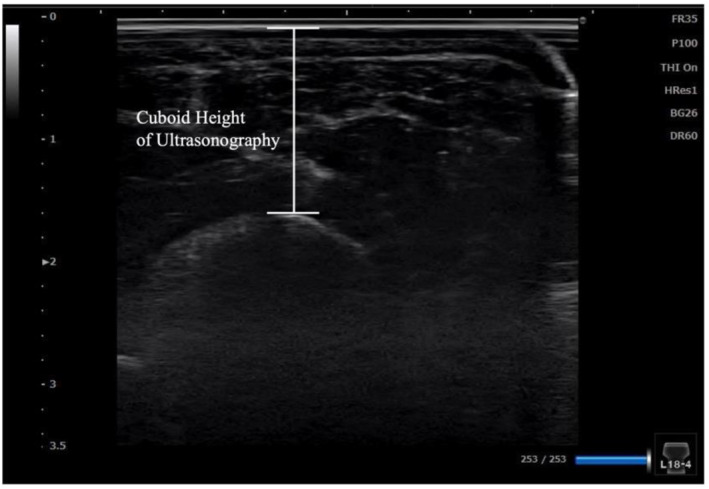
Calculation of the cuboid height using ultrasonography.
